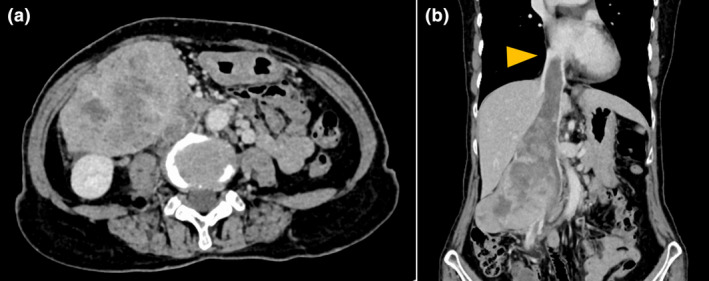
Contrast‐enhanced CT before surgery. (a) An axial view shows an 86 mm tumor in the right retroperitoneal space. (b) In a coronal view, the apex of the tumor thrombus (yellow arrowhead) extends into the right atrium.
